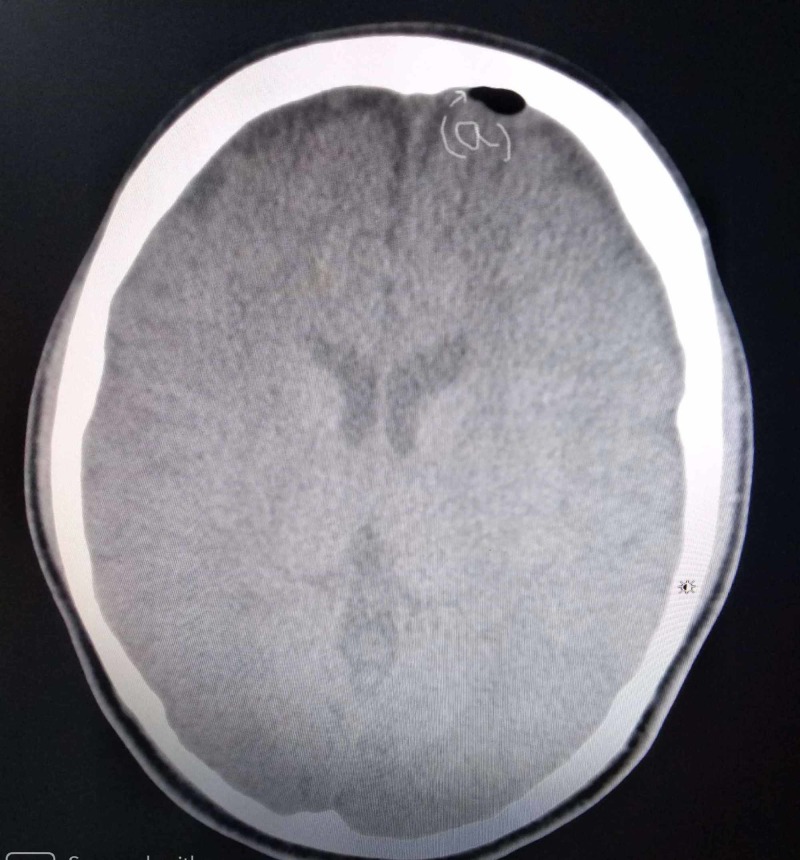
Axial CT scan, showing the increased size of pneumocephalus
